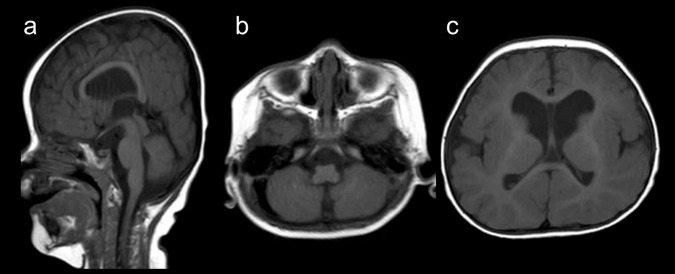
T1-weighted brain magnetic resonance imaging at 9 months. a A sagittal image shows brachycephaly and a thin corpus callosum. b, c Axial images show hypoplastic vermis of the cerebellum (b) and dilatation of the lateral ventricles associated with the cavum septum pellucidum.
